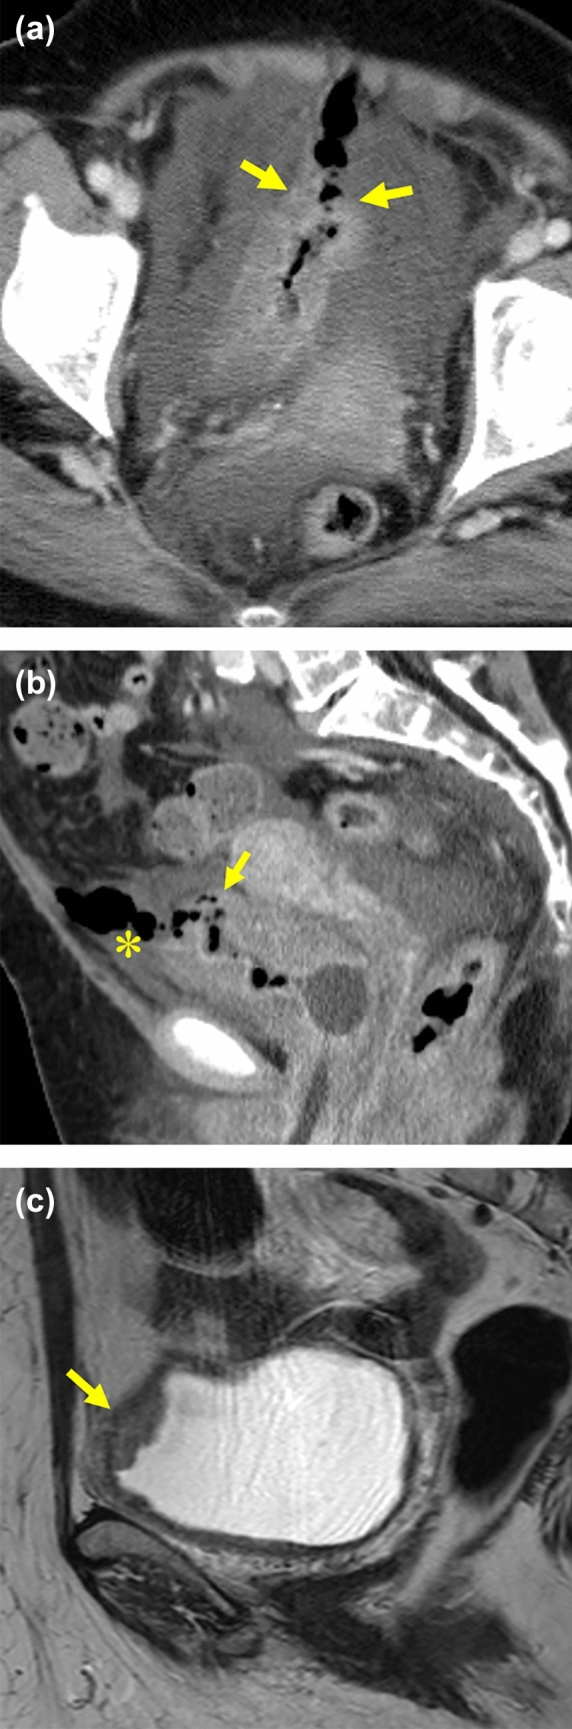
60-Year-old woman with bladder rupture complained abdominal pain the day after TURBT. a, b The axial a and sagittal b images of CECT show the tract-like gas at the bladder dome (arrows), retroperitoneal fluid collection with gas (in the paravesical space and Retzius cavity) (*) and moderate amount of ascites. c T2-weighted MR image before TURBT shows bladder tumor matching with the ruptured point (arrow)
